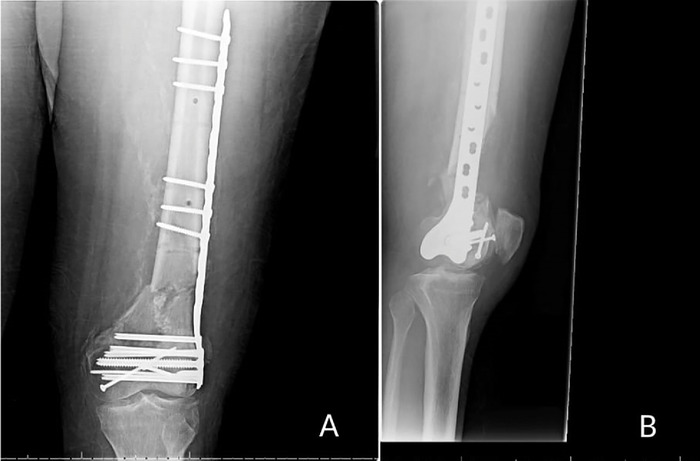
(A) Anteroposterior and (B) lateral radiographic films show the left femur following the first reconstruction after the motor vehicle collision in 2012.
